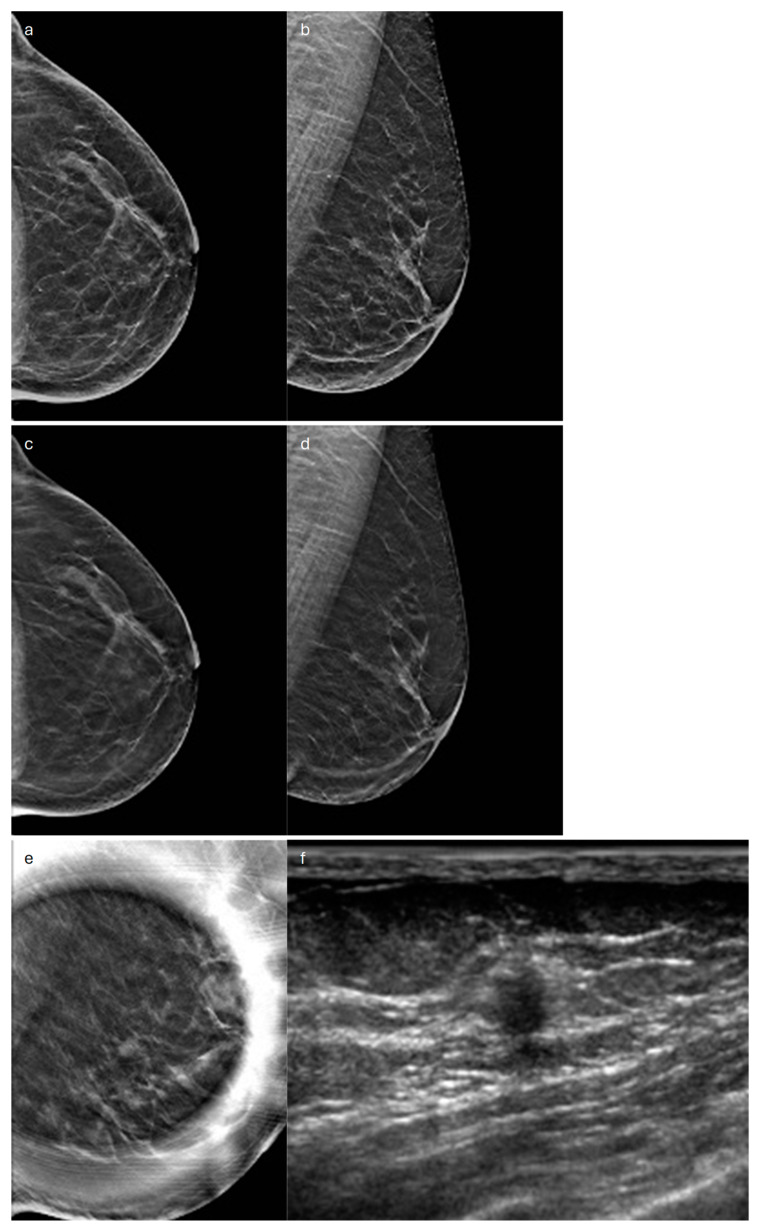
Tubular carcinoma. (a) Cranio-caudal and (b) medio-lateral oblique 2D synthetic mammograms (c), cranio-caudal and (d) medio-lateral oblique DBT images, and (e) spot compression view of the left breast showing a small opacity (f) corresponding to a hypoechoic mass with non-circumscribed margins on ultrasound.
